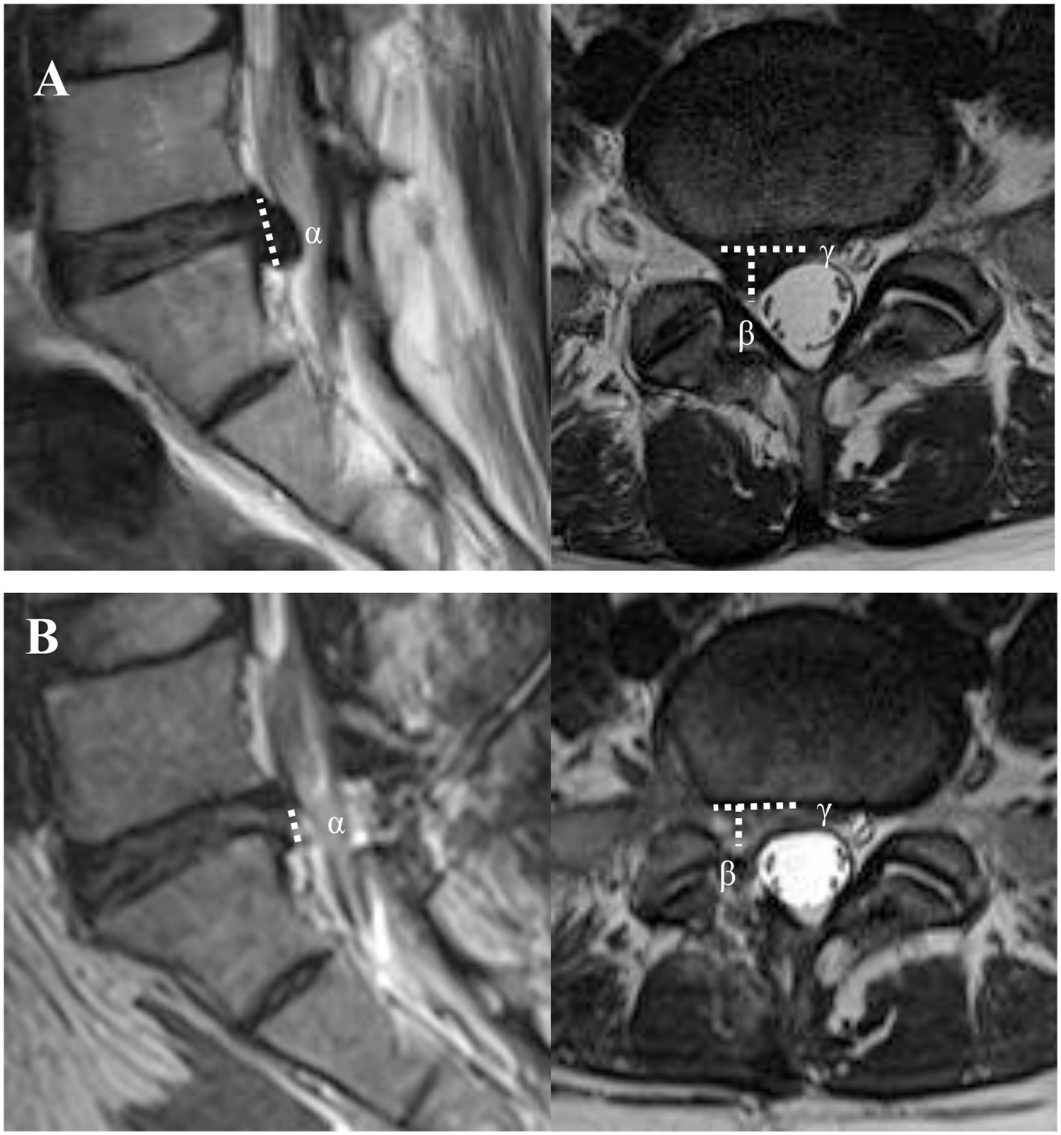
Assessment of the ruptured disc volume index (mm^3^) as the product of [height (α), length (β), and width (γ) recorded in (A) preoperative and (B) postoperative MRI scans. MRI: magnetic resonance imaging.
